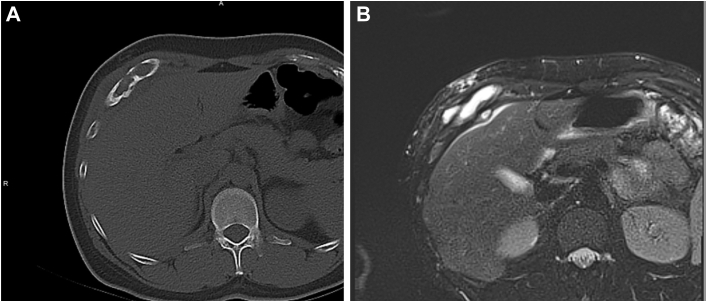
(A) Computed tomography of the thorax bone window. (B) T2-weighted magnetic resonance imaging of the chest wall.
